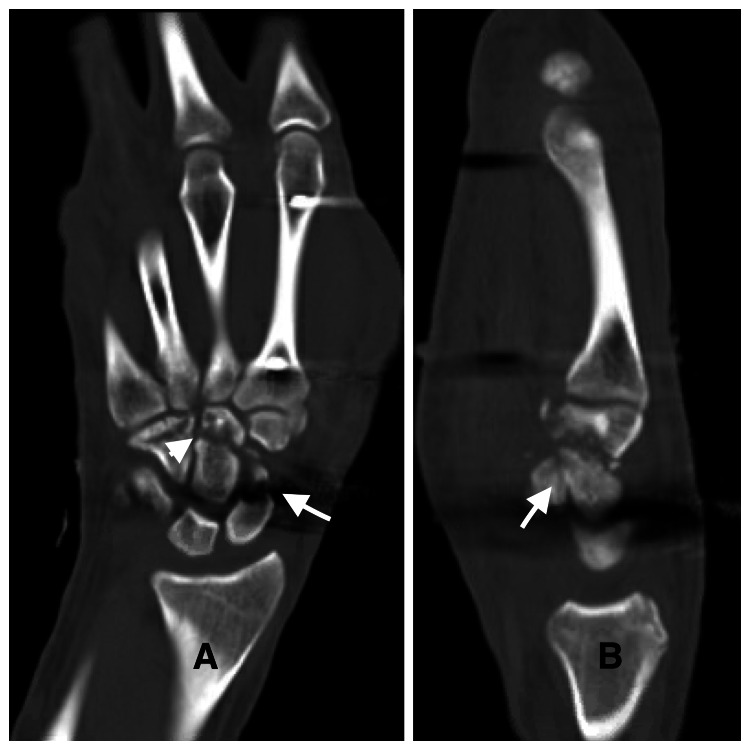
CT scan of right wrist showing right carpal bones fractures after external fixator application (A) Coronal and (B) sagittal reconstructed CT images (arrows show the scaphoid fracture, and the arrowhead shows the capitate fracture) CT: computed tomography
